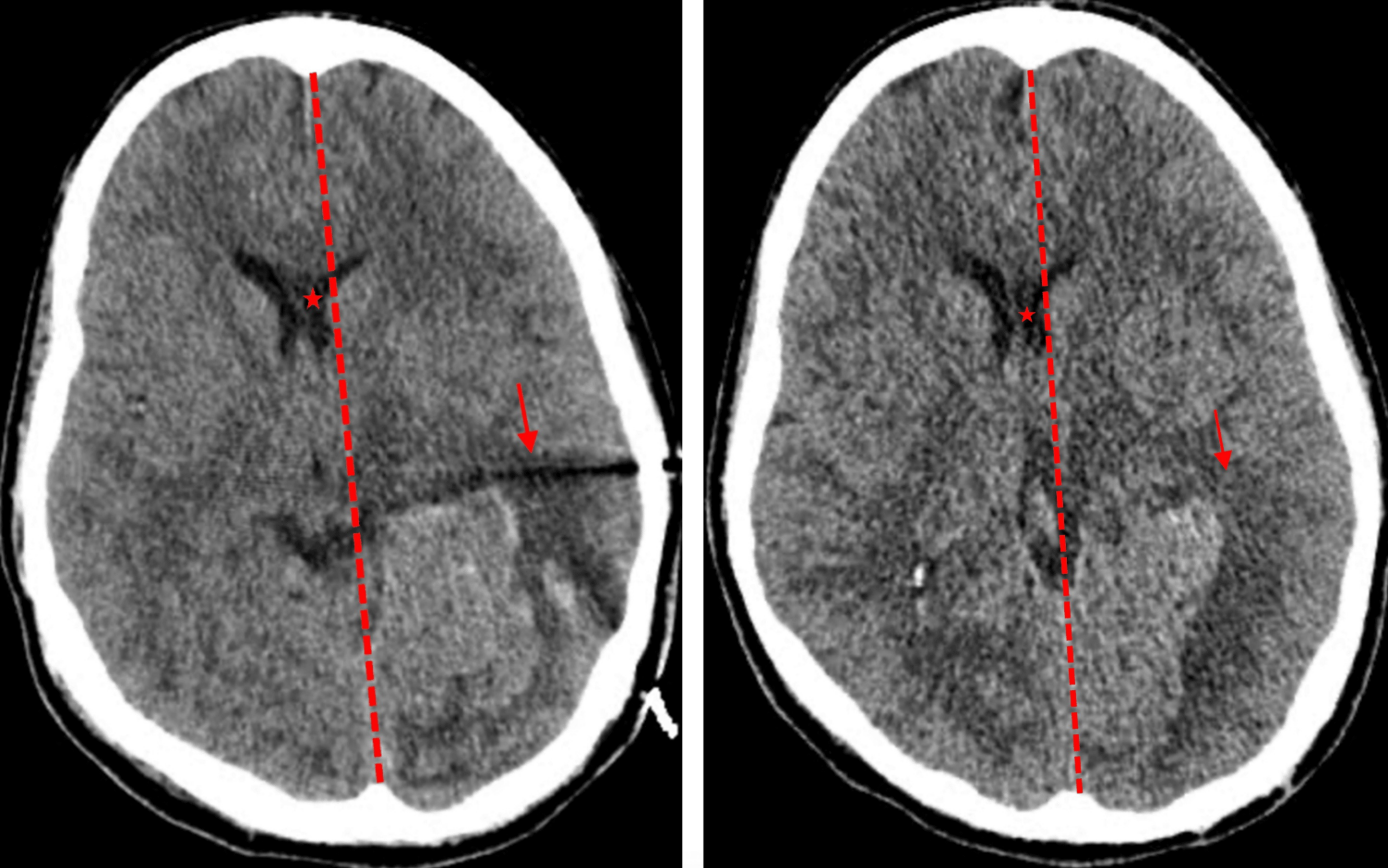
(A) Non-contrast brain CT; (B) subsequent non-contrast brain CT. The red arrow indicates a hypodense area in the left temporal lobe. The dashed red line indicates a midline shift toward the right. The red star depicts the rightward shift of the falx cerebri.
